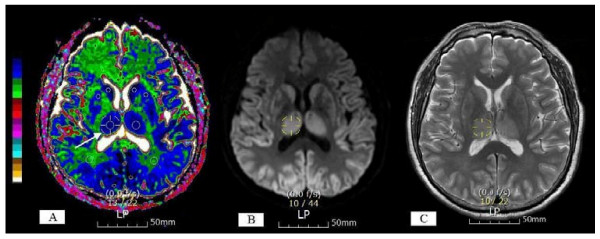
Apparent diffusion coefficient map with colour shades (A), diffusion-weighted imaging (B) and T2-weighted image (C) from one representative patient at seven hours after cardiac arrest. Regions of low apparent diffusion coefficient (ADC) showed a blue colour; in contrast, regions of high ADC showed a white colour. The colour shades on the ADC maps identified the pixel showing the minimum ADC value in each brain region. A 3D cursor (arrow) was used to select the predefined spot (right thalamus) simultaneously in the three different sequences, and it can be easy to mark the area with the minimum ADC on the ADC maps based on the T2-weighted image (T2WI) and diffusion-weighted imaging (DWI). The circular region-of-interest (ROI) cursors were positioned on the areas with the minimum ADC in each brain region. Severely restricted diffusion within the ROIs was shown in the caudate nucleus (0.238 × 10^-3^mm^2^/sec), putamen (0.299 × 10^-3^mm^2^/sec), thalamus (0.290 × 10^-3^mm^2^/sec), and occipital grey matter (0.184 × 10^-3^mm^2^/sec) but not in the occipital white matter (0.712 × 10^-3^mm^2^/sec).
